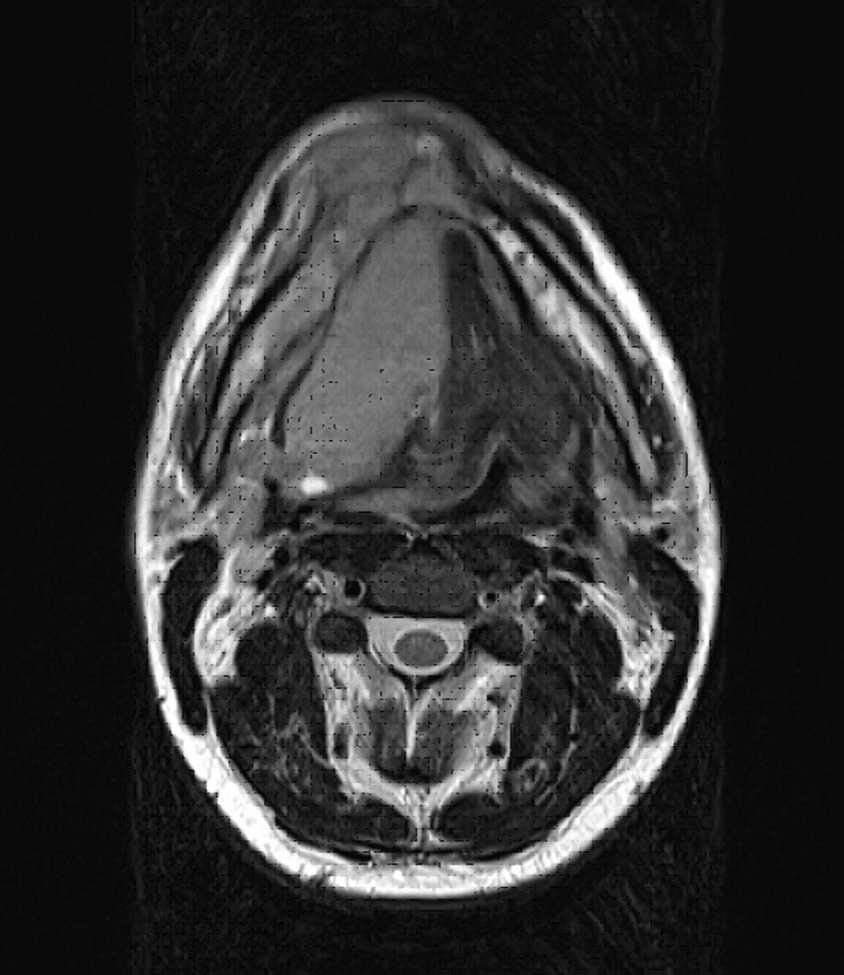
Axial MRI scan revealing a bone destroying mass of approximately 7 × 8 × 6 cm^3 ^surrounding the mandible and massively infiltrating the soft tissue of the floor of the mouth and the tongue.
